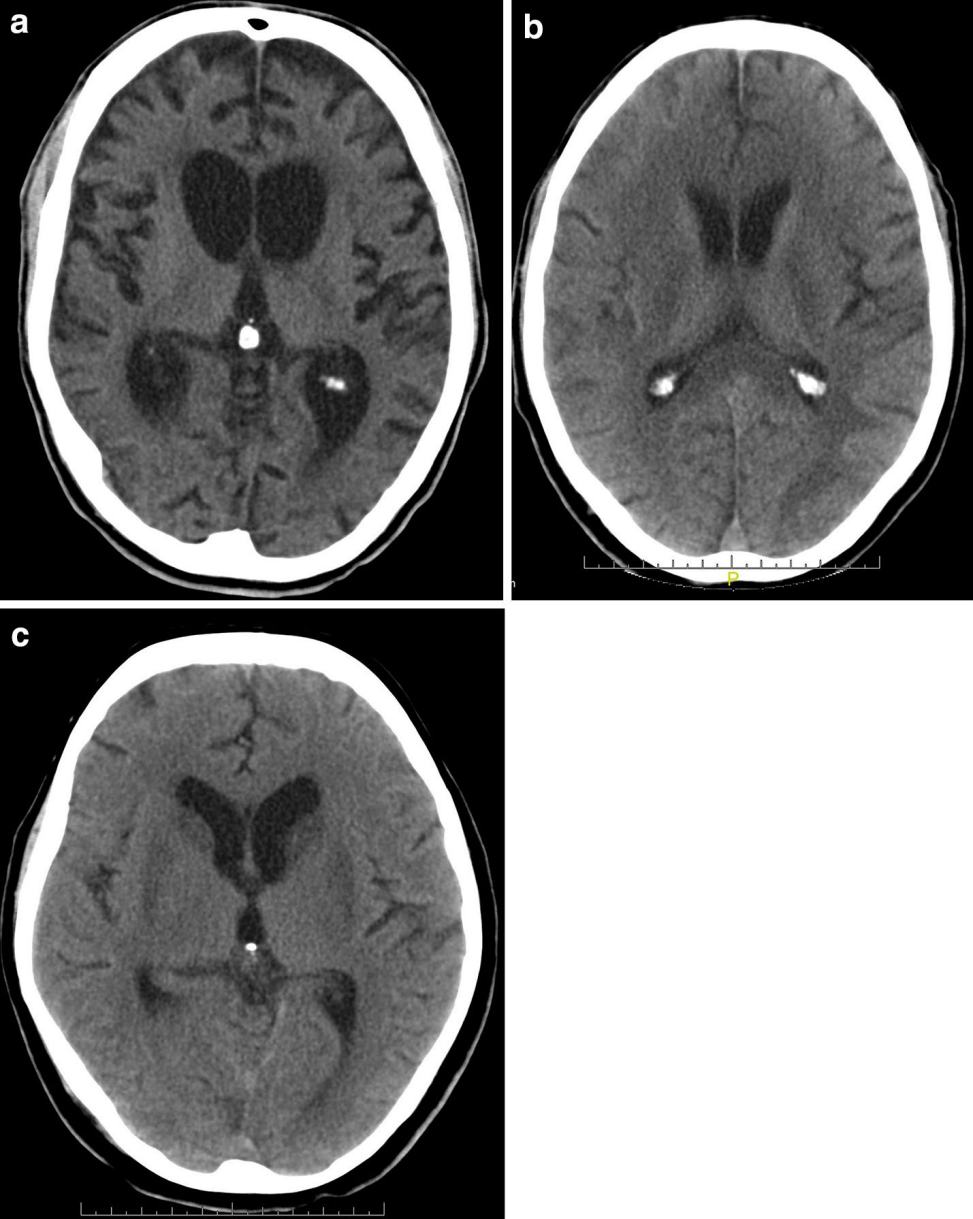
Typical CT scans of hypoxic brain damage patients. a Brain atrophy and hydrocephalus of a 44 y old male patient, 6 months after hypoxia. The patient was in a minimally conscious state (MCS). b Hypodense white matter changes of a 63 y old male patient, 2 weeks after hypoxic brain damage. The patient was in an unresponsive wakefulness syndrome (UWS). c Bilateral basal ganglia hypodensities of a 61 y old female patient, 6 weeks after hypoxic brain damage. The patient was in a MCS.
